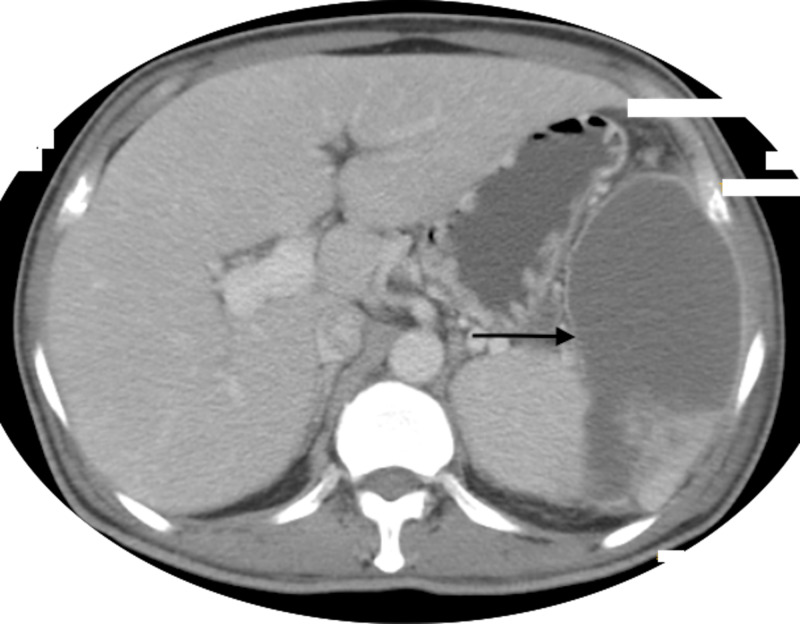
Contrast-enhanced CT of the abdomen showing splenic abscess (black arrow)
